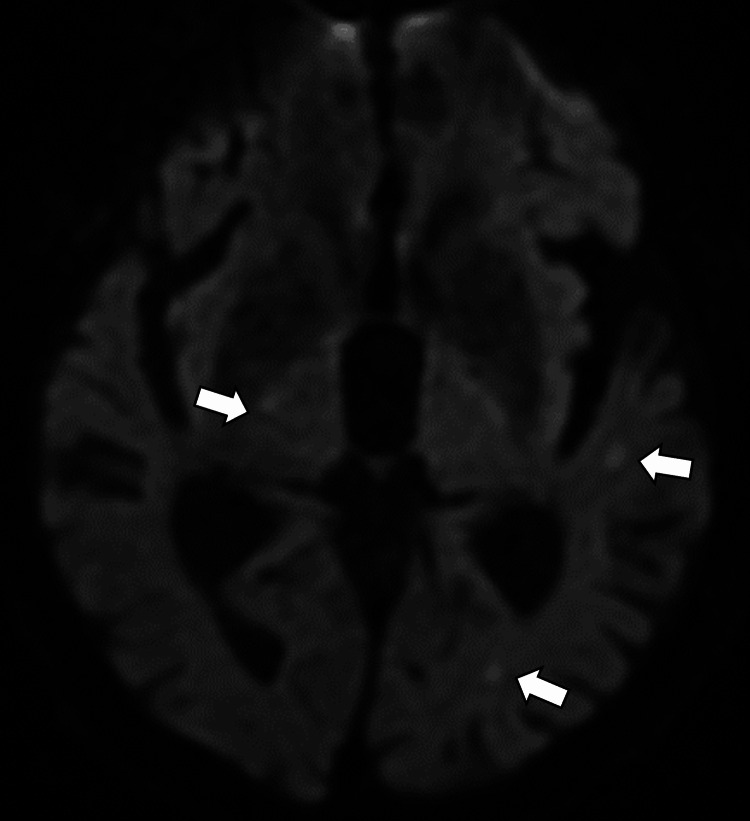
Brain magnetic resonance image revealing multiple hyperintense lesions on diffusion-weighted imaging (white arrows).
